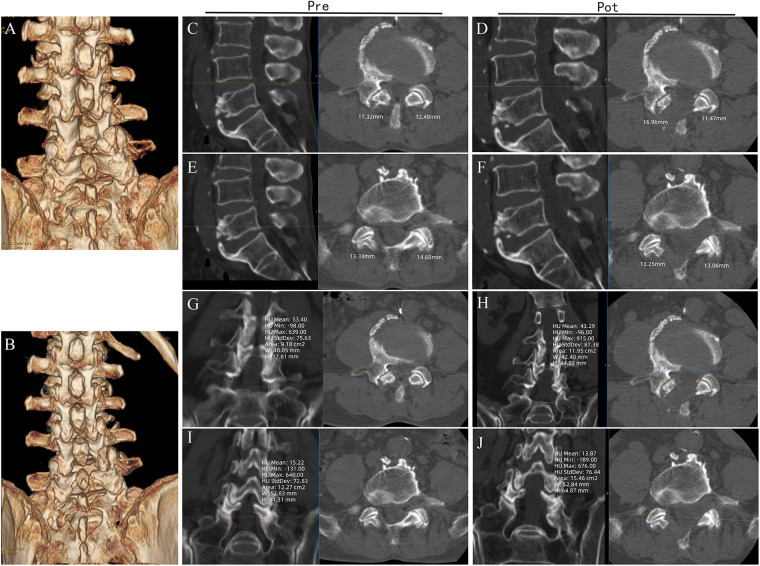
Preoperative vs. postoperative imaging. (A,B) 3D CT reconstruction: preop (A) and postope (B). (C,D) L3-4 sagittal and axial views: preop (C) and postop (D). (E,F) L4-5 sagittal and axial views: preop (E) and postop (F). (G,H) L3-4 coronal and axial views: preop (G) and postop (H). (I,J) L4-5 coronal and axial views: preop (I) and postop (J). Preop:preoperative. Postop:postoperative.
